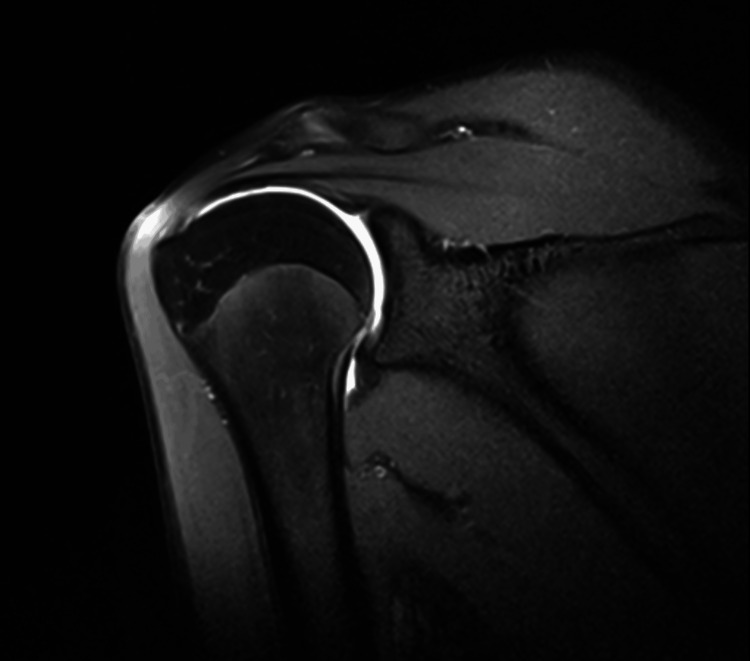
Coronal T1-weighted fat-suppressed MR arthrography image of the right shoulder demonstrating intra-articular contrast distribution with adequate capsular distension
